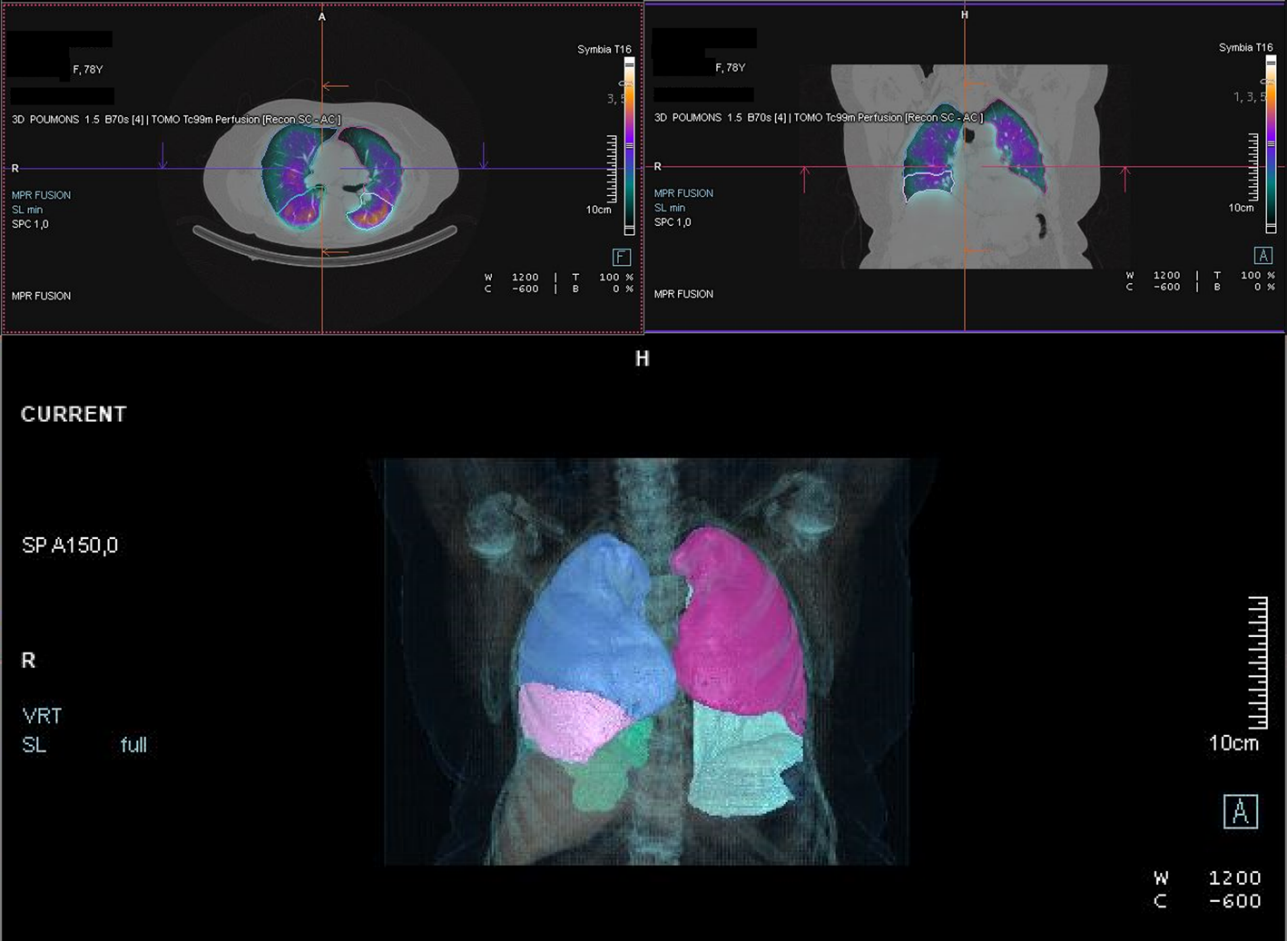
Automated lobar segmentation on the perfusion SPECT/CT dataset using the AI-based algorithm AutoLung3D. Representative data of a single patient (the same patient in Fig. 1) are shown
